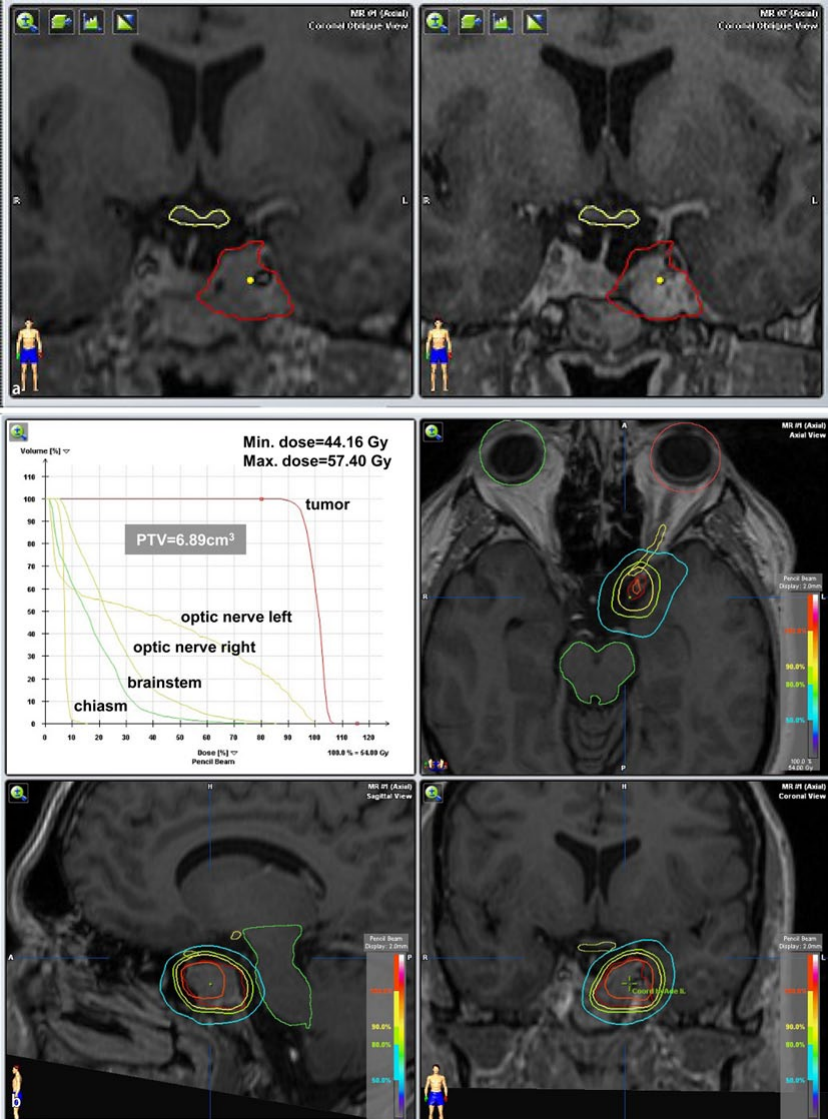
a, b MRI of patient (male, 41 years) with tumor progression after 2 transsphenoidal surgeries (the last in 2007), SRT with 5 × 1.8 ad 54 Gy in 2009 (CTV 5.13 ccm). At 3-year follow-up, tumor regression, no visual disorder, hypopituitarism (partial) idem
